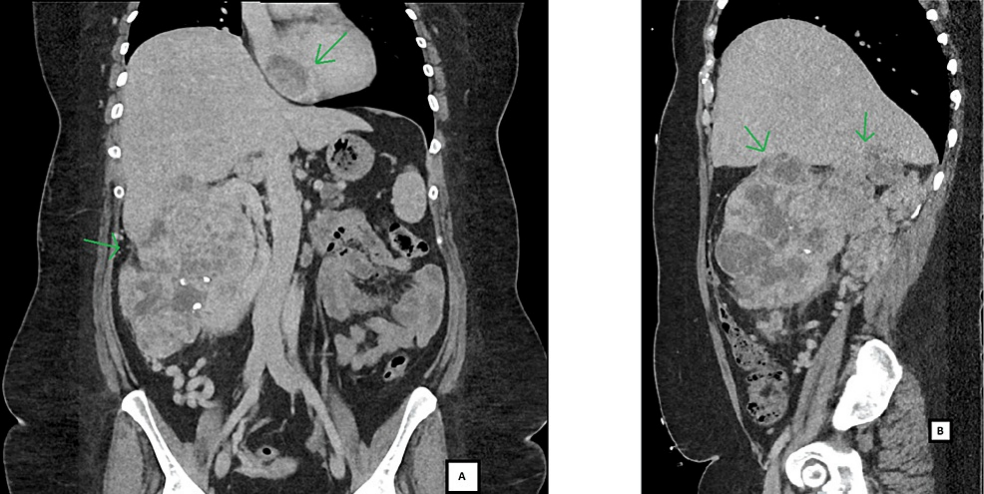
CT scan showing coronal (A) images of heterogenous mass with perinephric extension and right atrial lesion consistent with TT, and sagittal images (B) showing intrahepatic extension of mass. CT: computerised tomography, TT: tumour thrombus.
